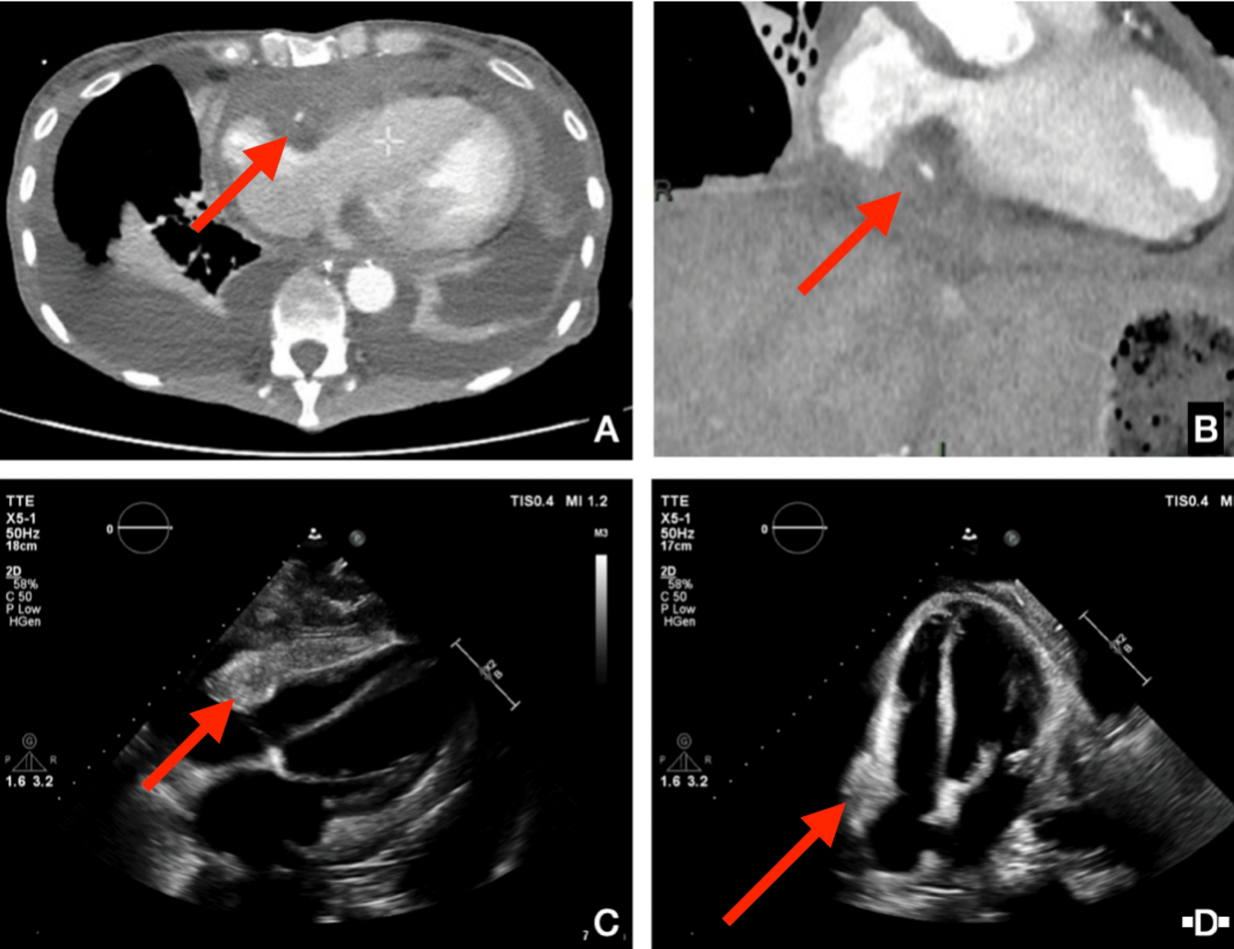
Imaging of constrictive pericarditis due to Whipple's disease A and B: Axial thoracic CT images, axial slice at the thoracoabdominal junction. The red arrows indicate a pericardial mass with mixed soft-tissue and fatty components encasing the right coronary artery. Additional signs of pericardial inflammation and bilateral pleural effusions are visible. Image B shows an enlarged view of the thickened pericardial tissue surrounding the coronary artery. C and D: Transthoracic echocardiography images demonstrating features of constrictive pericarditis. The red arrows highlight marked pericardial thickening (7 cm x 1.5 cm), particularly anterior to the right ventricle. Findings are consistent with a noncompliant pericardium restricting ventricular filling.
